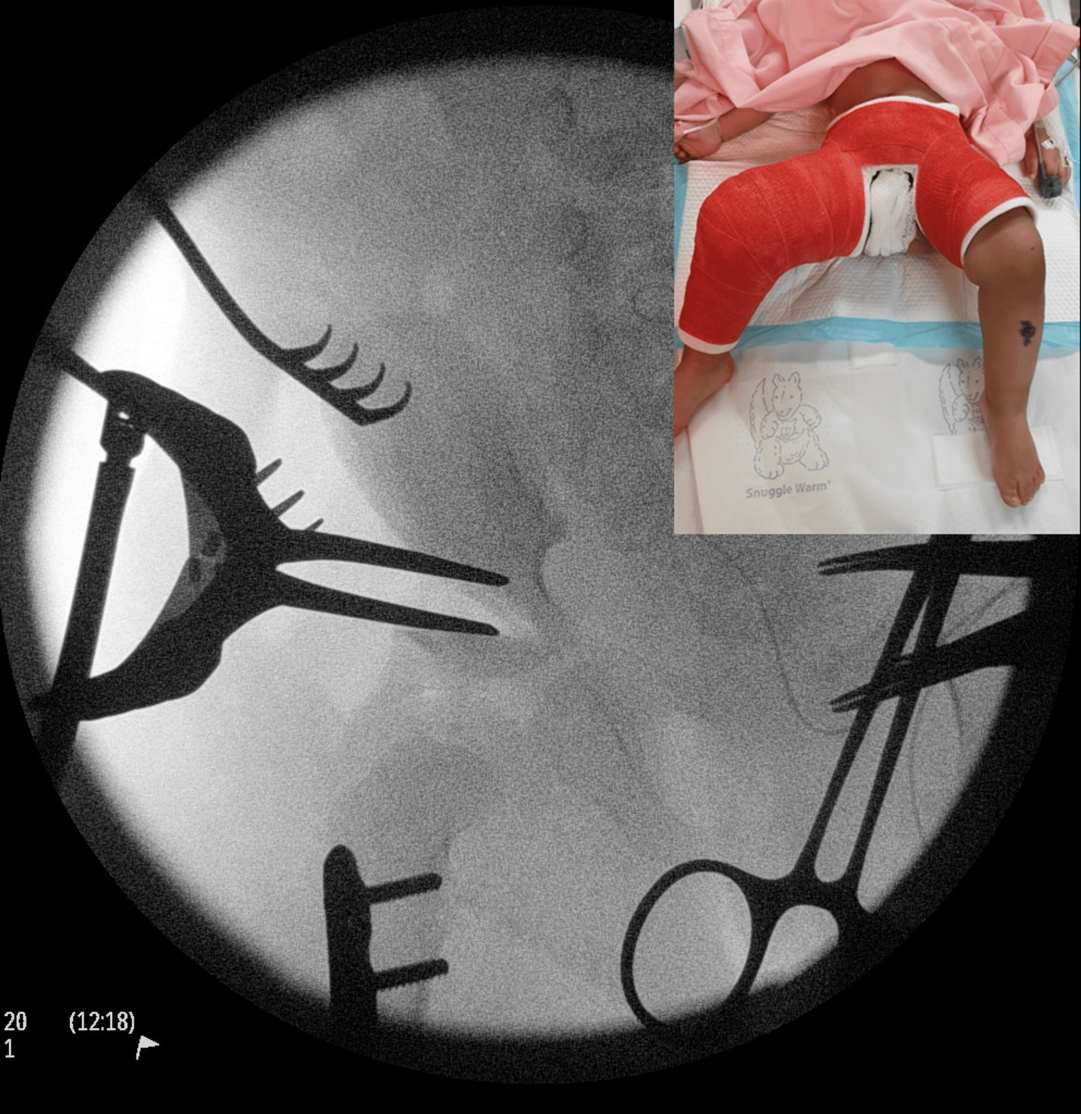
A compound image of open reduction of a dislocated hip in a child who presented late. Late presentation of hip dysplasia often involves major surgery of open reduction of the hip, pelvic, and femoral osteotomy. After surgery, the child is put in a hip spica (inset) for eight weeks. These could be prevented by early, high-quality hip ultrasound.
